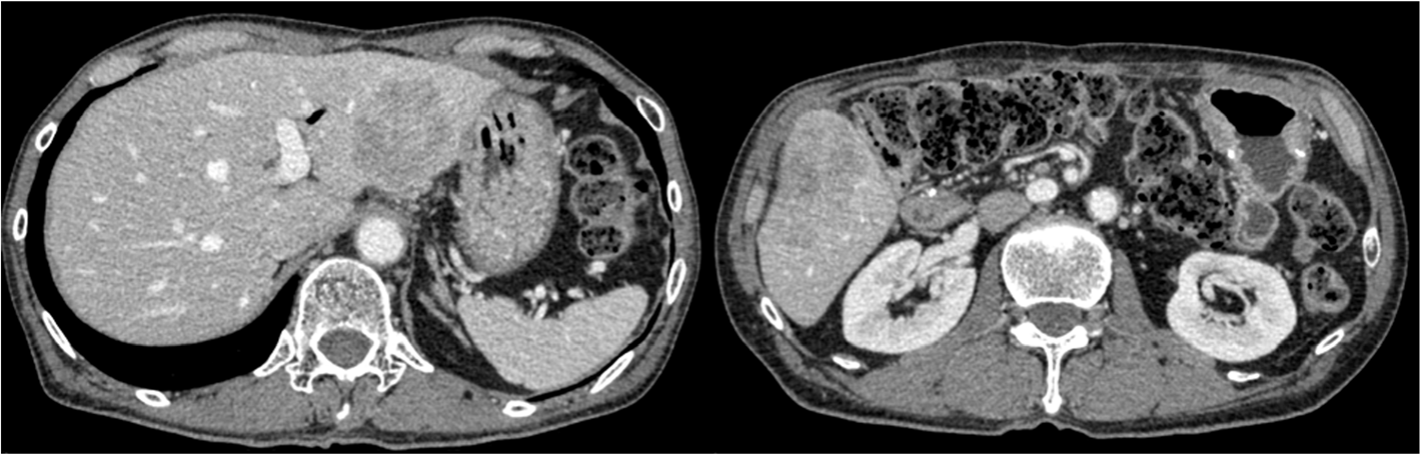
Abdominal contrast-enhanced CT scan image at the hepatic resection after chemotherapy showing growth of colorectal liver metastases (a, b)
